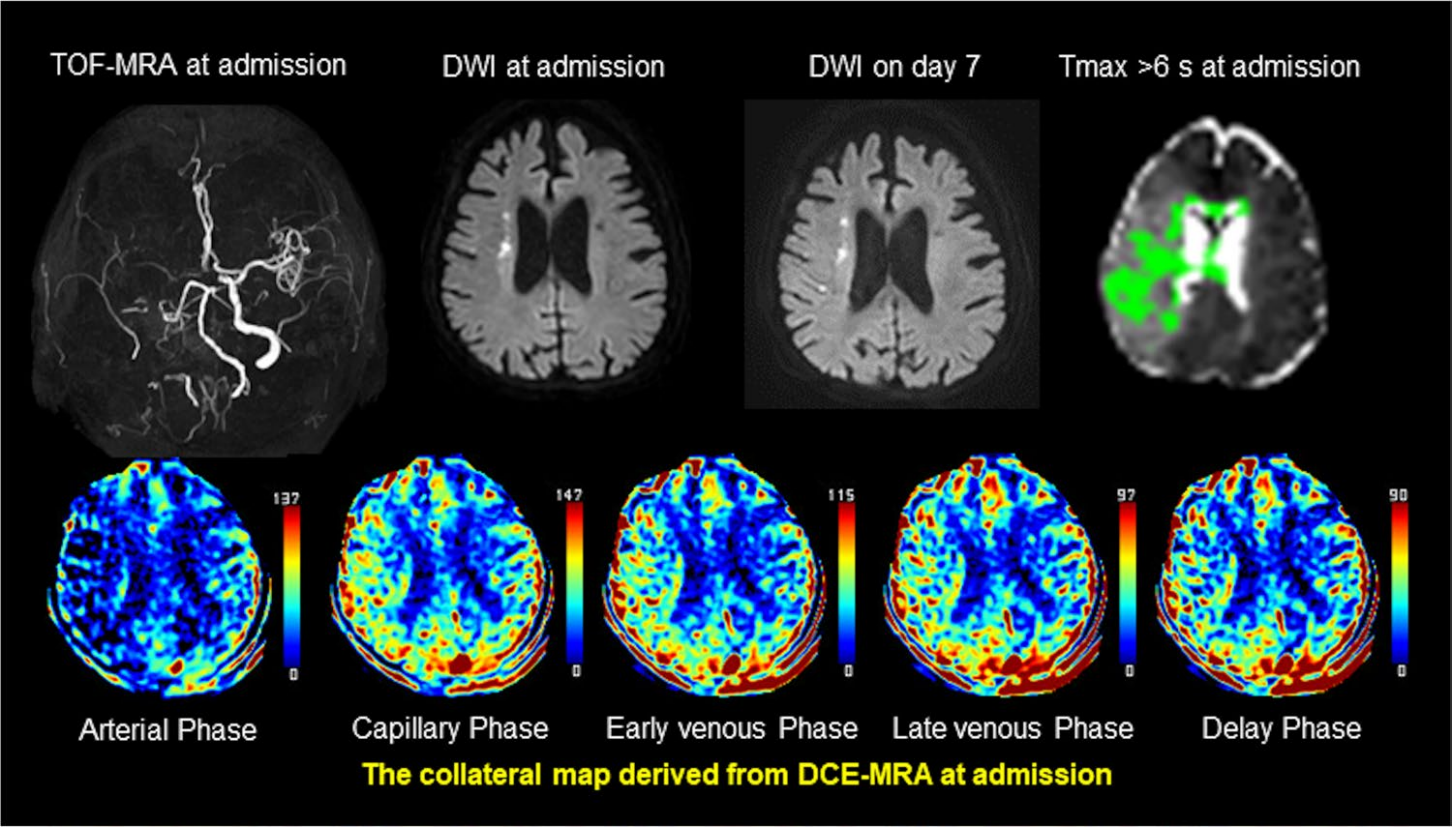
Case of good collateral perfusion grade. Images of an elderly patient with occlusion of the right internal carotid and middle cerebral arteries demonstrated on time-of-flight MR angiography (TOF-MRA). The premorbid modified Rankin scale score of this patient was 0, and the National Institutes of Health Stroke Scale score at admission was 5. The patient underwent intravenous thrombolysis followed by intraarterial thrombectomy, but recanalization of the occluded arteries was not achieved. Diffusion-weighted imaging (DWI) at admission showed acute infarction in the right middle cerebral artery territory. The collateral map derived from dynamic contrast-enhanced magnetic resonance angiography (DCE-MRA) at admission shows a good collateral perfusion status (MR acute ischemic stroke collateral perfusion score of 5: no collateral perfusion delay in the middle cerebral artery territory in the capillary phase). The baseline DWI lesion did not grow significantly on DWI on day 7. The phase_baseline and phase_FU cannot be determined in the collateral map because there is no hypoperfused lesion approximate to the baseline and follow-up DWI lesions on the collateral map. An MR perfusion image with a threshold of  > 6 s of the contralateral time-to-maximum (Tmax > 6 s) shows a large penumbra (green area). Tmax > 6 s overestimates the final infarct extent
